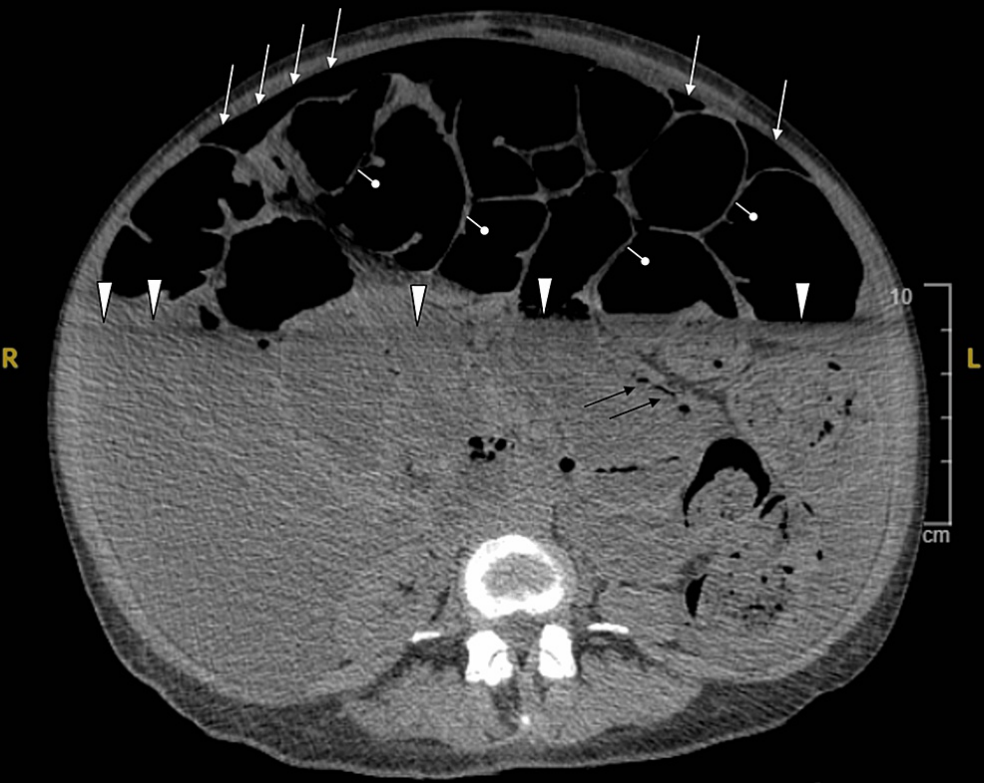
CT of the abdomen without contrast, supine position, displaying PI (black arrows), diffuse ascites (white arrowheads), and PI (black arrows), atrophy of intestinal villi (pinheads), and pneumoperitoneum (white arrows). PI - pneumatosis intestinalis
